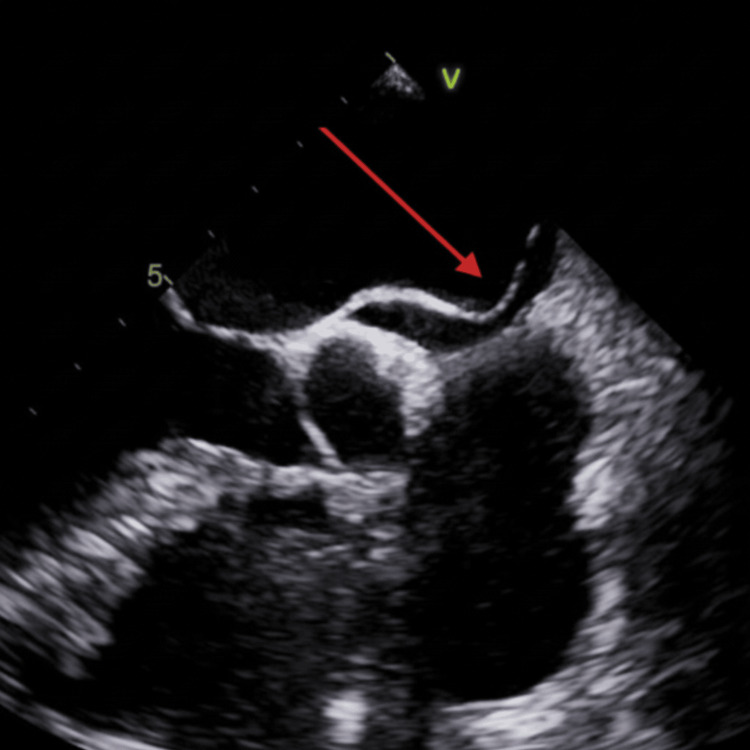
TEE Demonstrating Atrial Septal Aneurysm TEE image demonstrating an aneurysmal interatrial septum with septal deviation toward the right atrium, with the red arrow indicating the aneurysm. TEE: transesophageal echocardiography
